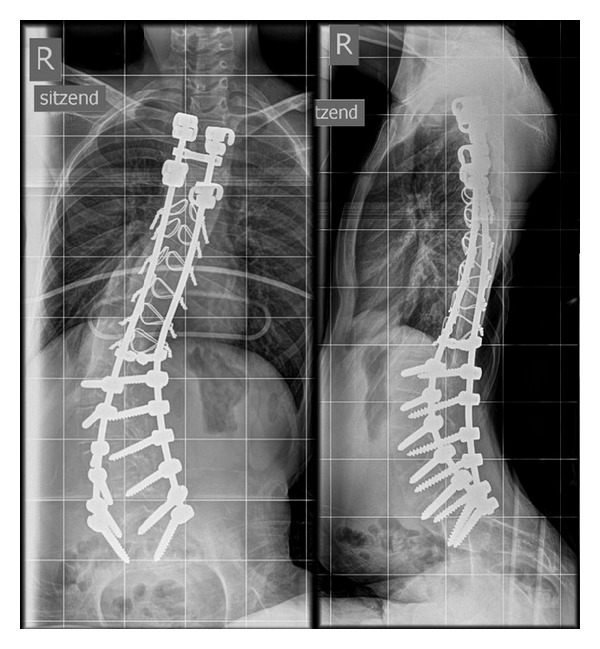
Male PD patient, 13 years old. Thoracic scoliosis 35° Cobb Th6/L1/L5, after surgical spine stabilization. Remaining 8° of pelvic obliquity to the right. AP and sagittal radiograph.
